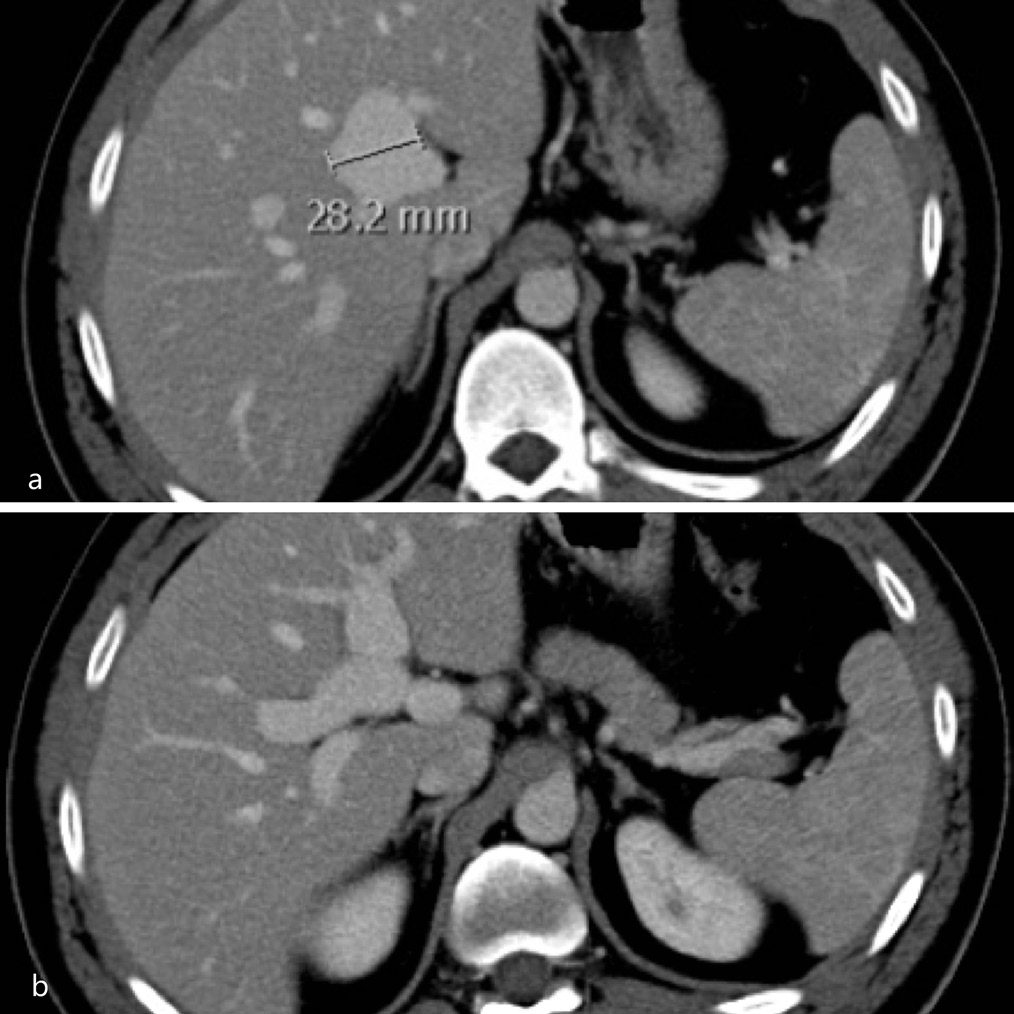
(a, b). Enhanced CT abdomen in portal venous phase demonstrated an aneurysmal dilatation involving the confluence of the portal vein extending into the left main portal vein. The maximum diameter at the confluence measures 2.8 cm.
